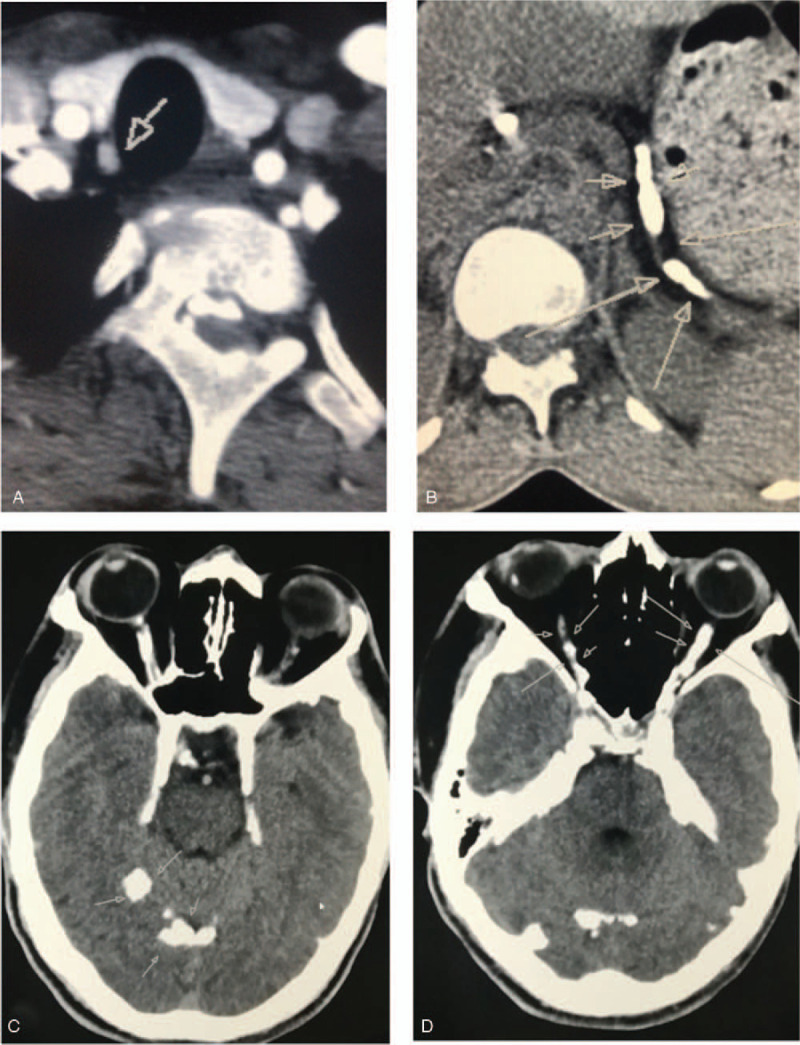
Some of the proband's radiograph findings. (A) Thyroid-enhanced CT suggested a suspicious nodule below the right thyroid gland; (B) Pancreas-enhanced CT suggested pancreatic atrophy and multiple stones in the pancreatic duct; (C and D) Head CT suggested extensive meningeal calcification including tentorium cerebelli, cerebral falx, and bilateral optic nerve calcification.
